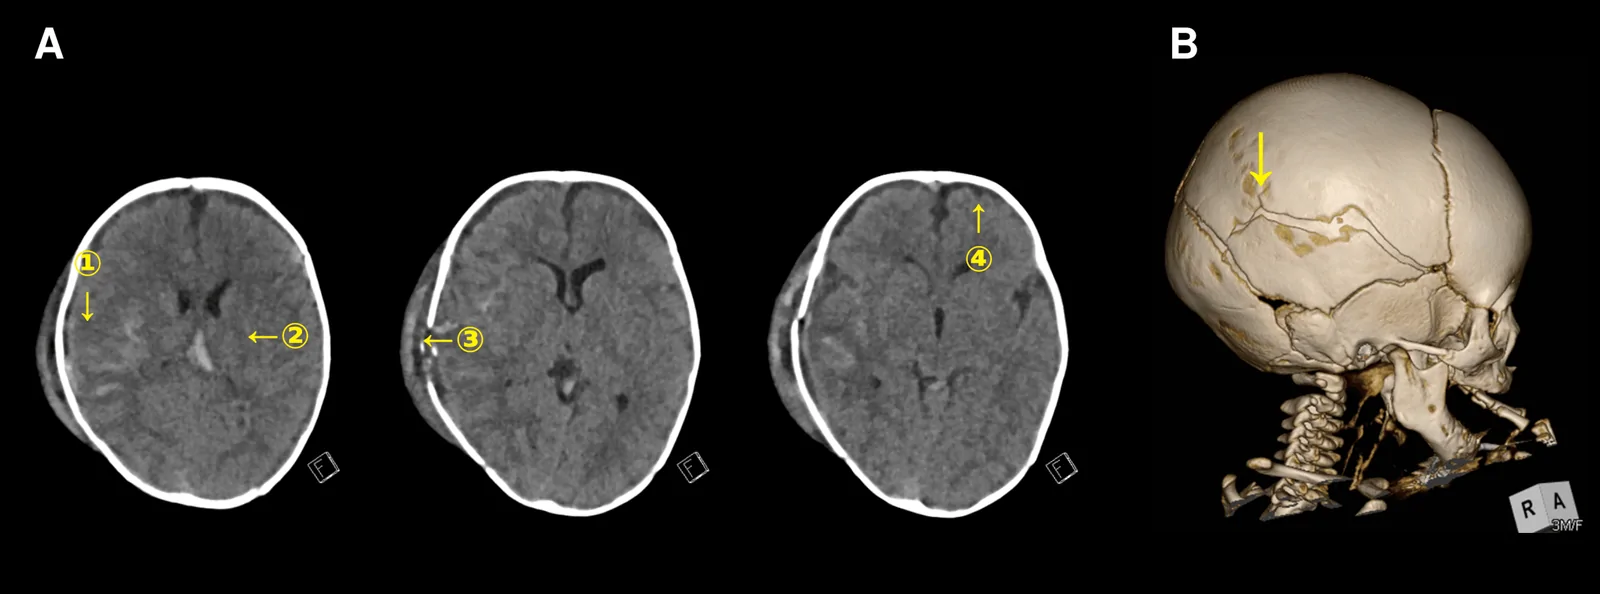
Initial head computed tomography (CT) obtained at 1 h post-injury (A) Axial CT images revealing associated traumatic intracranial injuries, including (1) right temporoparietal contusion with traumatic subarachnoid hemorrhage, (2) intraventricular hemorrhage, (3) acute subdural hematoma in the right temporal region, and (4) left temporoparietal contusion. (B) Three-dimensional reconstructed CT image demonstrating a right temporoparietal skull fracture with diastatic separation (arrow).
